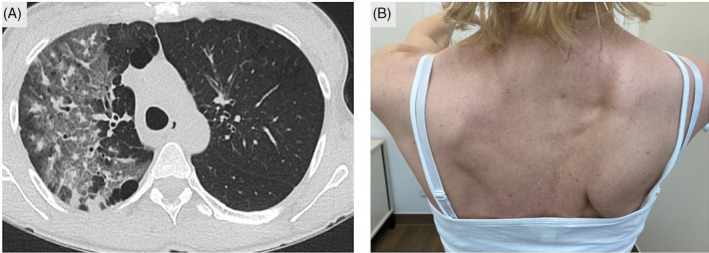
(A) Computed tomography showing extensive ground glass opacities and small, patchy consolidations in the upper lobe of the right lung. (B) Right‐sided amyotrophy of dorsal shoulder muscles and winged scapula (scapula alata).
